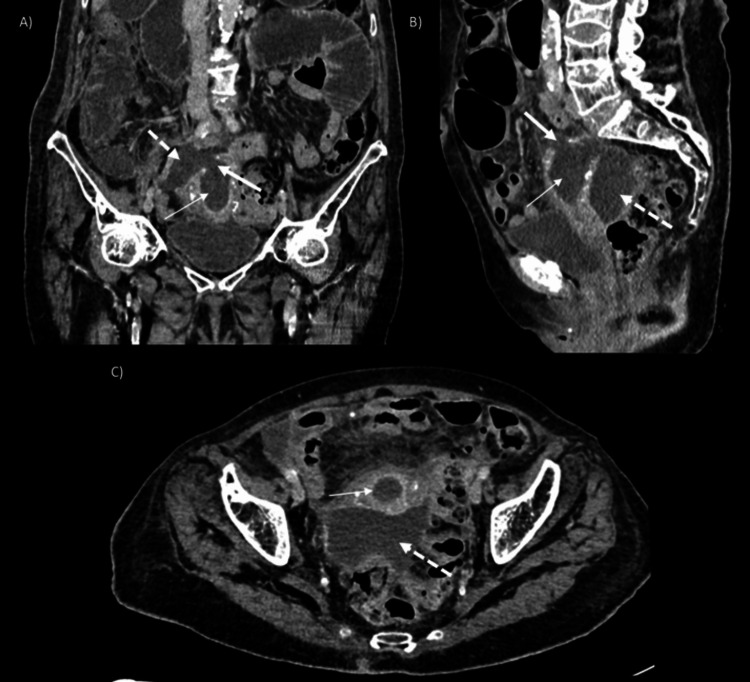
Computerized tomography in the venous phase of the abdomen. Coronal (A), sagittal (B) and axial (C) CT scans show distension of the endometrial cavity (thin arrow) with a 1.5-cm diameter defect in the uterine wall at the fundus (thick arrow) communicating with an abscess in the pouch of Douglas (dotted arrow).
